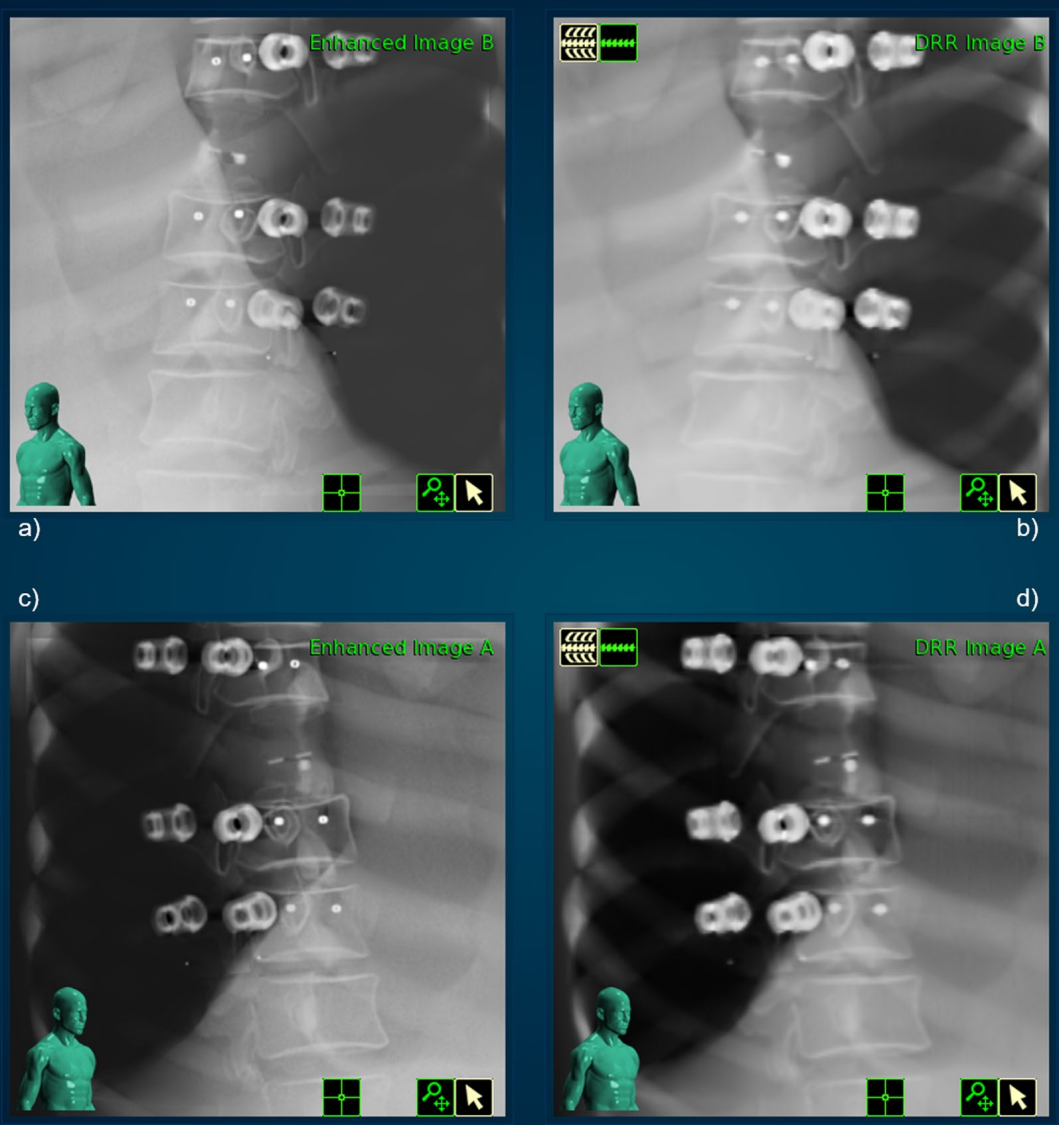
Live image for both x-ray cameras on a, c as well as the corresponding calculated DDR images on b, d
